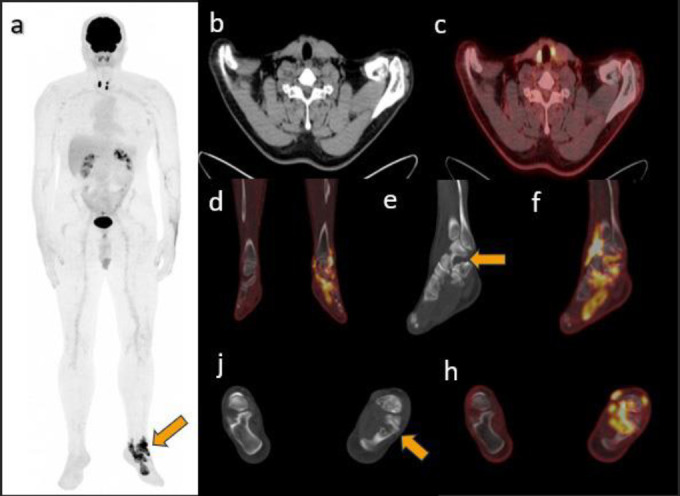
a. Maximum imaging projection (MIP), b-c. CT and fused images showing intense FDG uptake in thyroid bed, and d-h. Infiltrative soft tissue lesions involving left ankle and ventral surface of the foot with multiple lytic bone lesions (thick arrow)
